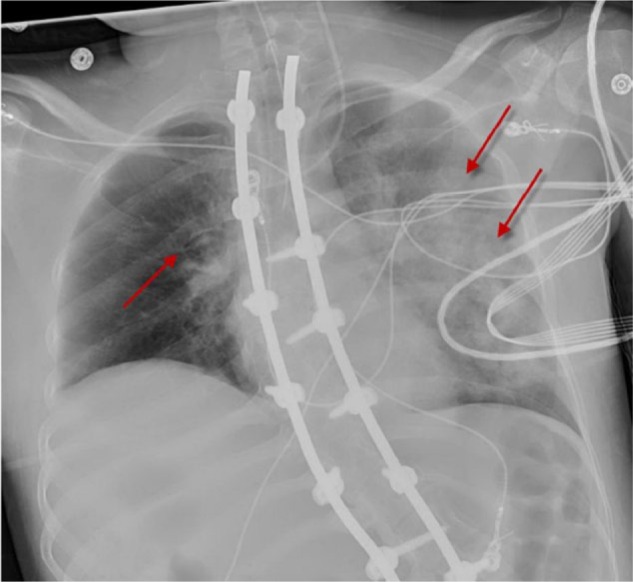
Chest radiograph demonstrating diffuse bilateral infiltrates (left > right) and marked pulmonary vascular congestion (arrows).
